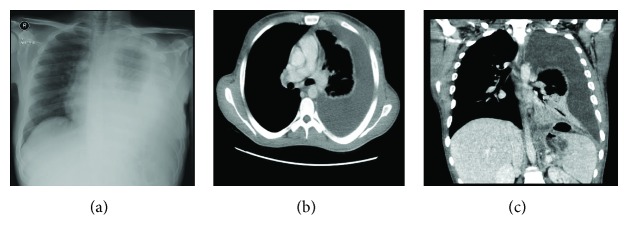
(a) Posterio-anterior chest radiograph showing a large left pleural effusion with atelectasis of the left lung. (b) Axial chest CT showing a large left pleural effusion with complete atelectasis of the left lower lobe. (c) Sagittal abdominal and pelvis CT showing a large leak with perisplenic collection. The abdominal collection is connected to the pleural collection through a fistula.
